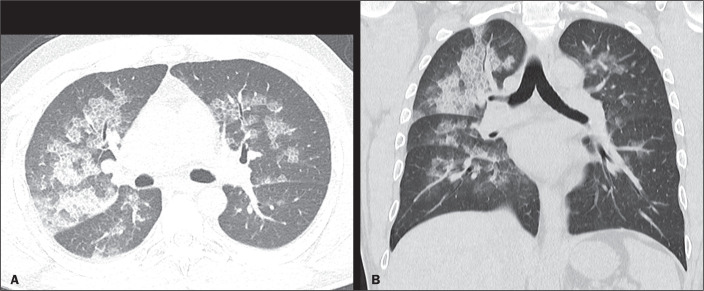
A 44-year-old man with dyspnea after extubation. Computed tomography
